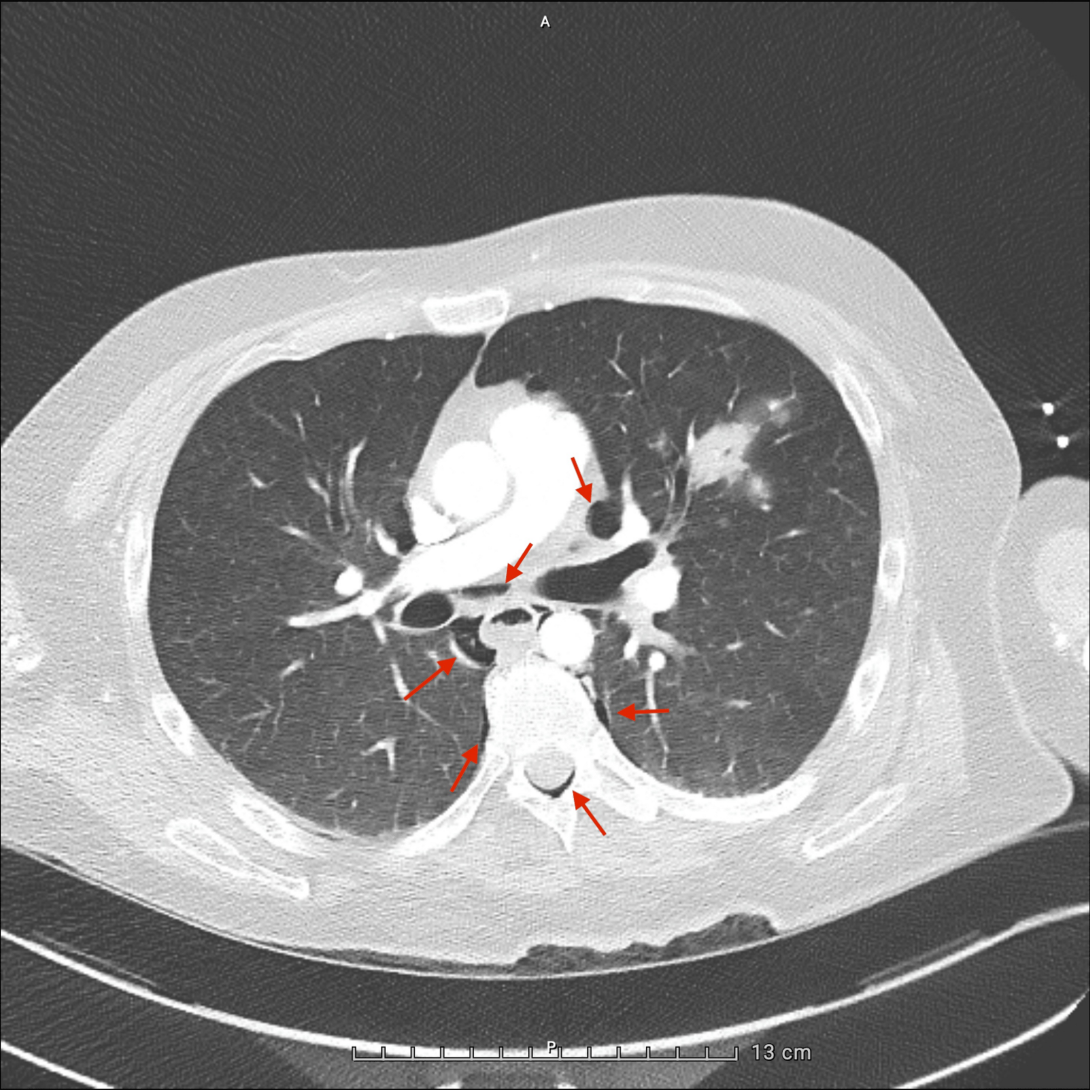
Axial chest CT – mid thoracic level slice showing pneumomediastinum and epidural air. A patchy consolidation is also noted in the left upper lobe, likely reflecting alveolar filling in the context of aspiration or prolonged immobilization.
